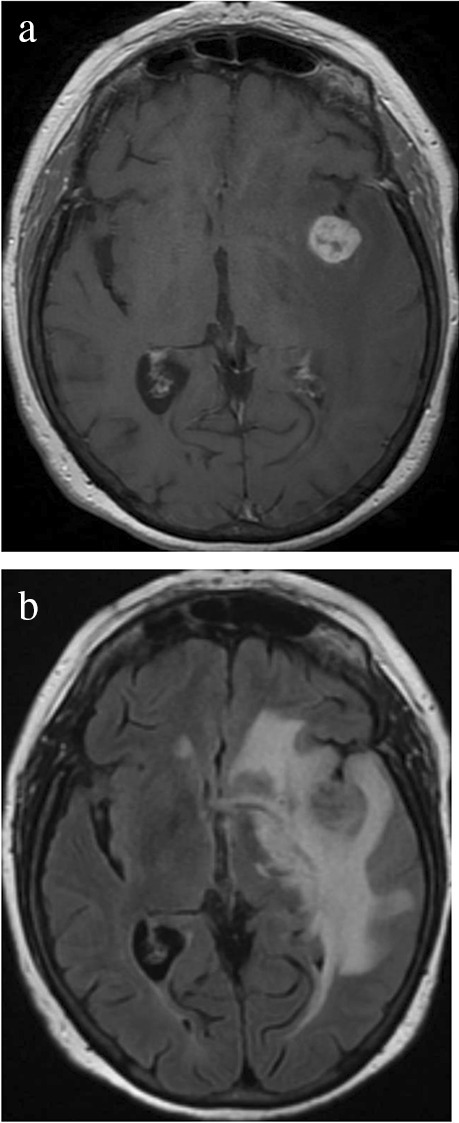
T1-weighted magnetic resonance image shows a left-sided, well-circumscribed contrast-enhancing lesion measuring 2 cm in diameter (a). T2-weighted FLAIR image (fluid attenuated inversion recovery) reveals marked surrounding edema (b)
